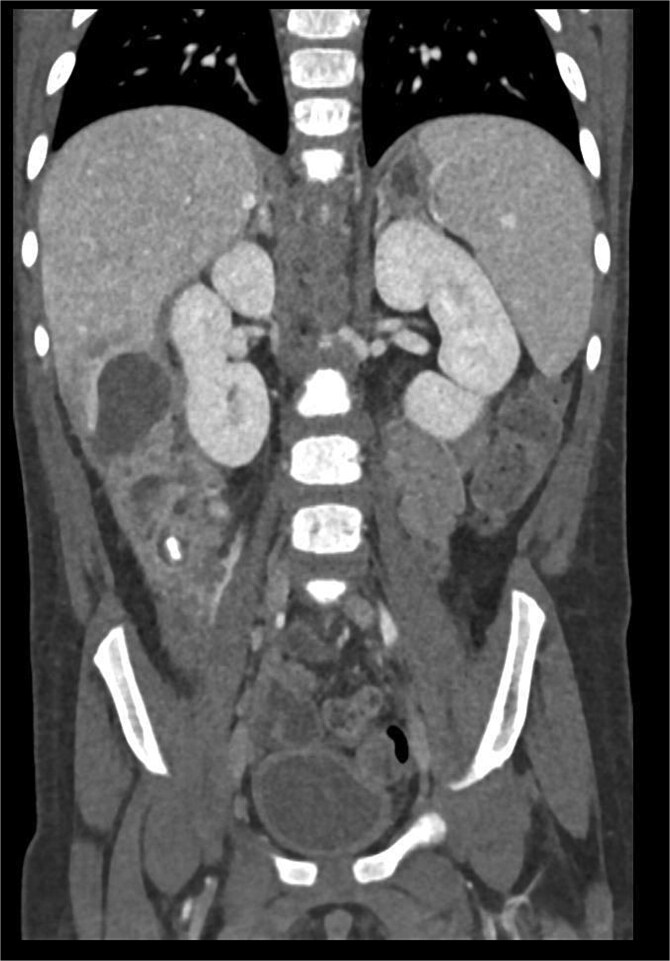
CT imaging of acute appendicitis with fecalith and multiple hepatic abscesses.
